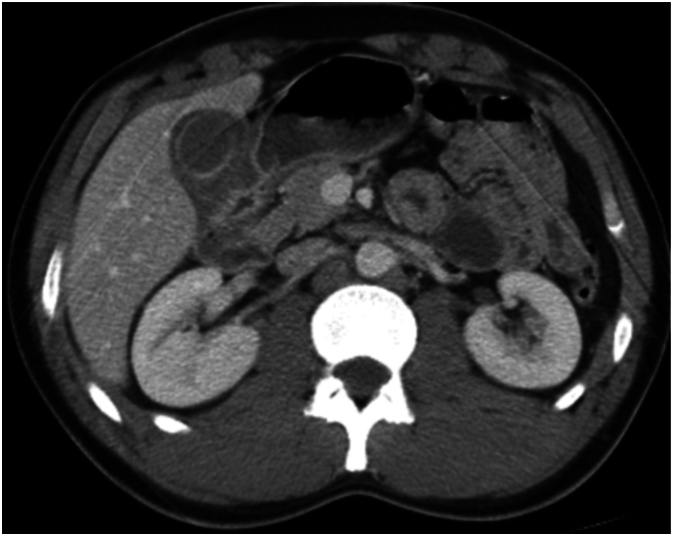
Computed tomography showing a thickened gallbladder wall, a significant amount of pericholecystic fluid, and thickening of gastric and duodenal walls.
